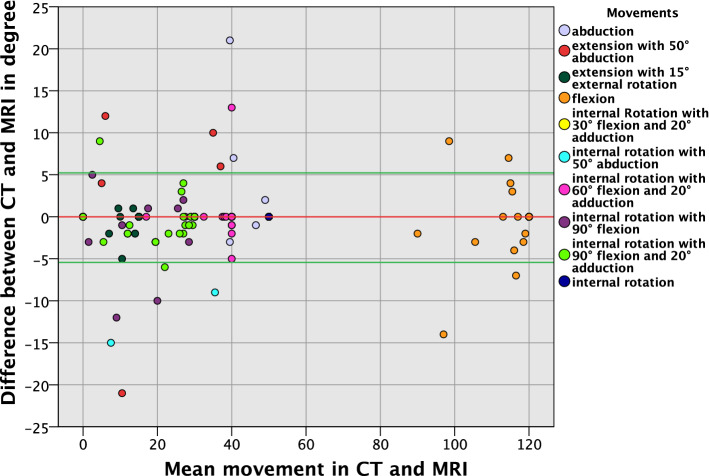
Bland––Altman plot for all ROM analyses displaying differences between MRI- and CT-derived measurements; red line—mean; green line—1.96 standard deviation (light blue—abduction; red—extension with 50° abduction; dark green—extension with 15° external rotation, orange—flexion, yellow—internal rotation with 30° flexion and 20° adduction, turquoise—internal rotation with 50° abduction, pink—internal rotation with 60° flexion and 20° adduction, violet—internal rotation with 90° flexion, light green—internal rotation with 90° flexion and 20° adduction, dark blue—internal rotation)
